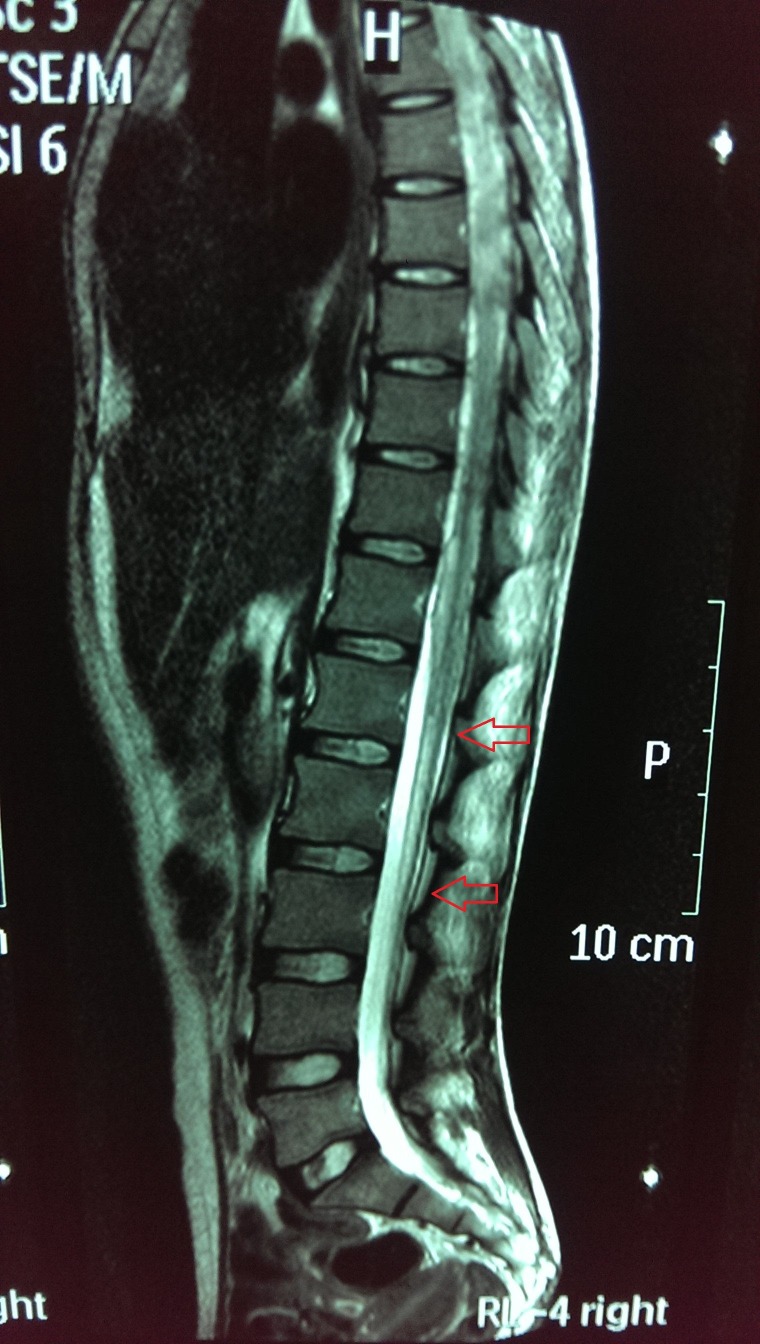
Dorsolumbar magnetic resonance imaging (MRI) spine with contrast Arrows representing syrinx formation. The cord appears low lying showng a fusiform T2W abnormal hyperintense signal area in its distal part extending from D12 to L3.
